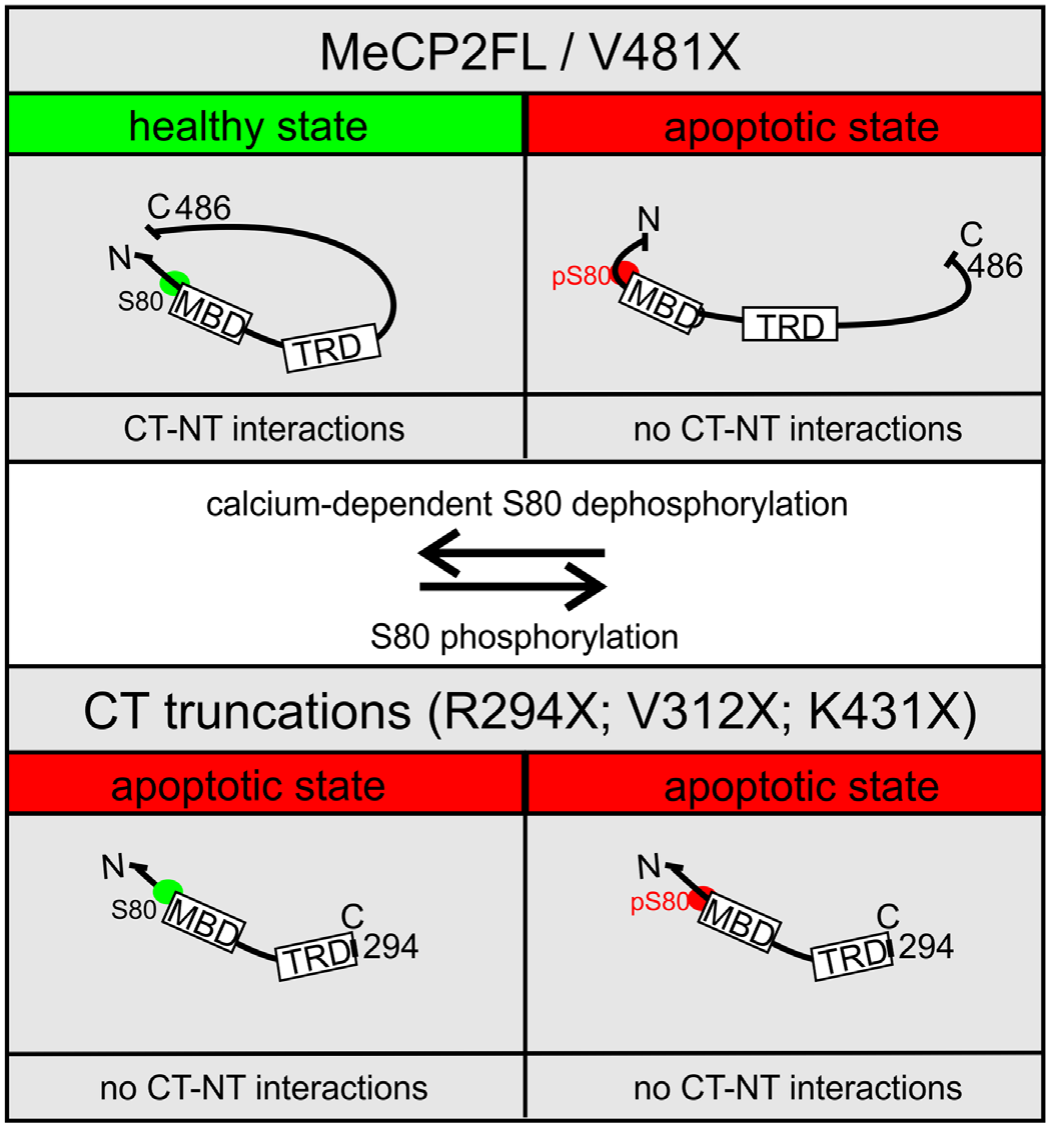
Working model. Phosphorylation of MeCP2FL at S80 induces conformational changes of MeCP2 that prevent C- and N-terminal interactions (CT-NT interactions), thus inducing conformational changes that (apoptotic state, red) underlie the apoptotic effect of MeCP2 *in vivo*. Calcium dependent de-phosphorylation at S80 returns MeCP2 to a healthy conformation (green) that allows CT-NT interactions. C-terminal truncation prevents CT-NT interactions thus favoring the apoptotic conformation of MeCP2. R249X is shown as an example, but it is expected that V312X and K431X truncations act in the same manner. The short truncation V481X removes just 5 amino acids, which does not prevent CT-NT interactions thus favoring the healthy conformation of MeCP2.
